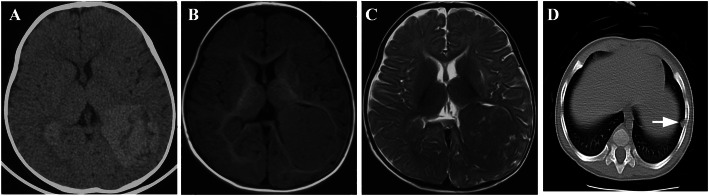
a Brain CT shows multiple punctate, flaky, and round-like high-density holes in the bilateral lateral ventricles and bilateral subependymal. A large lesion located in the posterior corner of the left lateral ventricle was diagnosed as SEGA. b-c Head MRI shows nodules in the posterior corners of bilateral ventricles on T1- and T2-weighted images. d Chest CT showed pulmonary subpleural nodule (white arrow)
